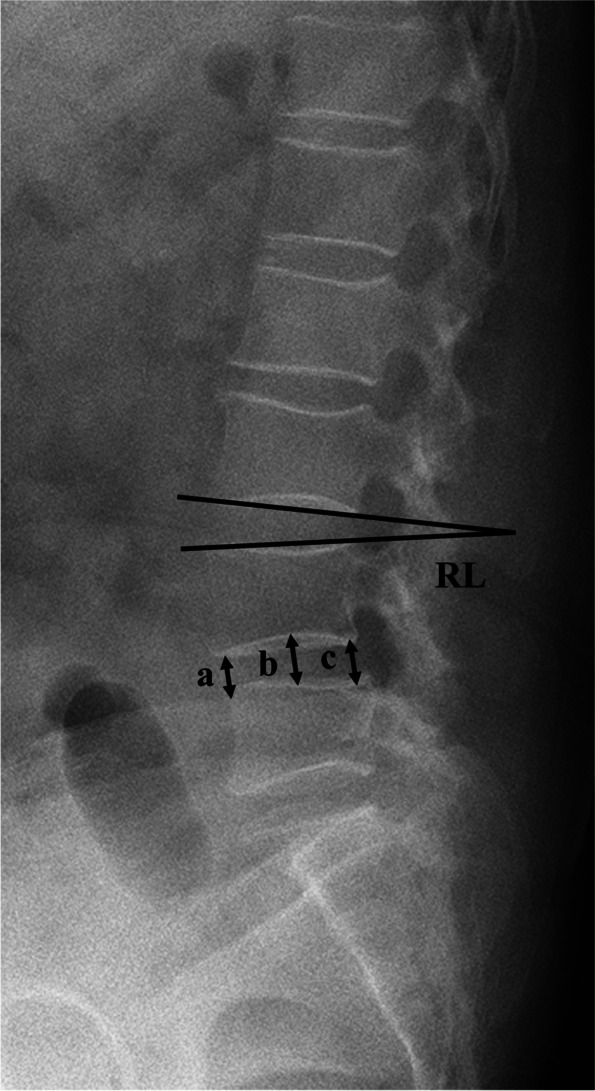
Radiographic measurements: RL (regional lordosis), the angle between the upper and lower edges of the intervertebral disc; DH (disc height), the mean of the anterior (a), middle (b), and posterior disc height values (c)
